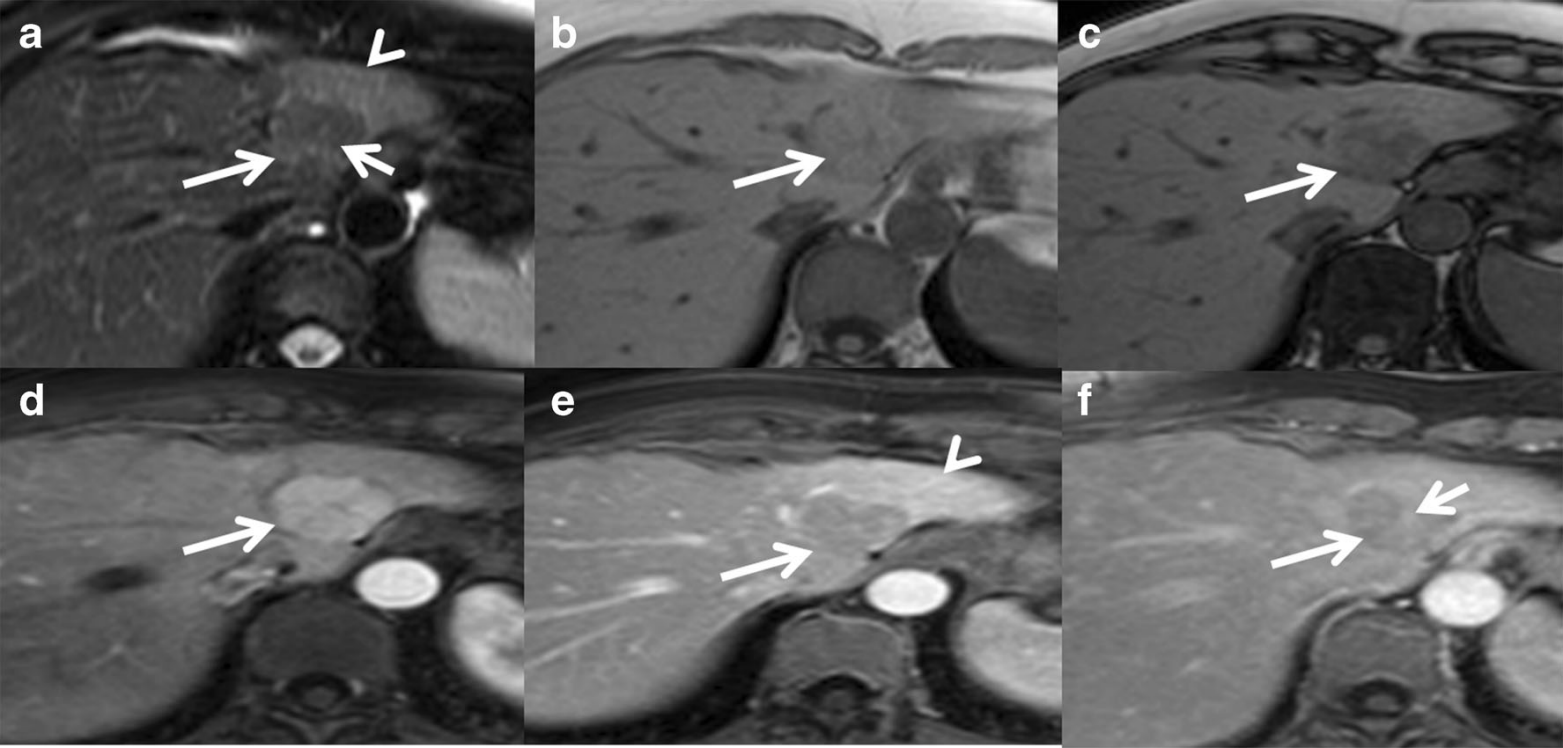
Biopsy-proven FNH with intralesional steatosis in a 46-year-old female. (a) Axial T2W MRI shows an isointense lesion (*long arrow*) in the left lobe of the liver with a faint hyperintense central scar (*short arrow*). Axial T1 in-phase (b) and out-of-phase (c) MRIs show a drop in signal in the out-of-phase images representing intralesional lipid (arrow). Axial post-contrast MRI shows an enhancing lesion (*arrow*) in the arterial phase (d) becoming iso- to mildly hypointense in the portal venous (e) and delayed phases (f) with delayed enhancement of the central scar (*arrow*). Arrowheads denote transient oedema/enhancementTelangiectatic FNH is an uncommon entity but the most common among non-classic FNHs. These lesions often show heterogeneity on pre- and post-contrast CT and MRI, and they are strongly hyperintense on T1- and T2-weighted images, often with an absent central scar. Intense arterial enhancement with persistent enhancement during the hepatic venous and equilibrium phases is seen. Recently, a molecular study identified several genetic similarities between telangiectatic FNH and HCA indicating most of these are actually inflammatory hepatic adenomas and have been misclassified as 'telangiectatic FNH' [30].Development of FNH in childhood and presence of symptoms can also be considered as atypical features.Approximately 20 % of patients have multiple FNHs, which are often of a non-classic subtype with atypical imaging features. Multiple FNH syndrome is defined as consisting of two or more FNHs in combination with hepatic liver haemangioma or vascular malformations or intracranial tumours [31, 32] (Fig. 5). Focal disturbance of the hepatic blood supply has been advocated as the most likely causative factor for the concomitant development of these benign hepatic lesions.
